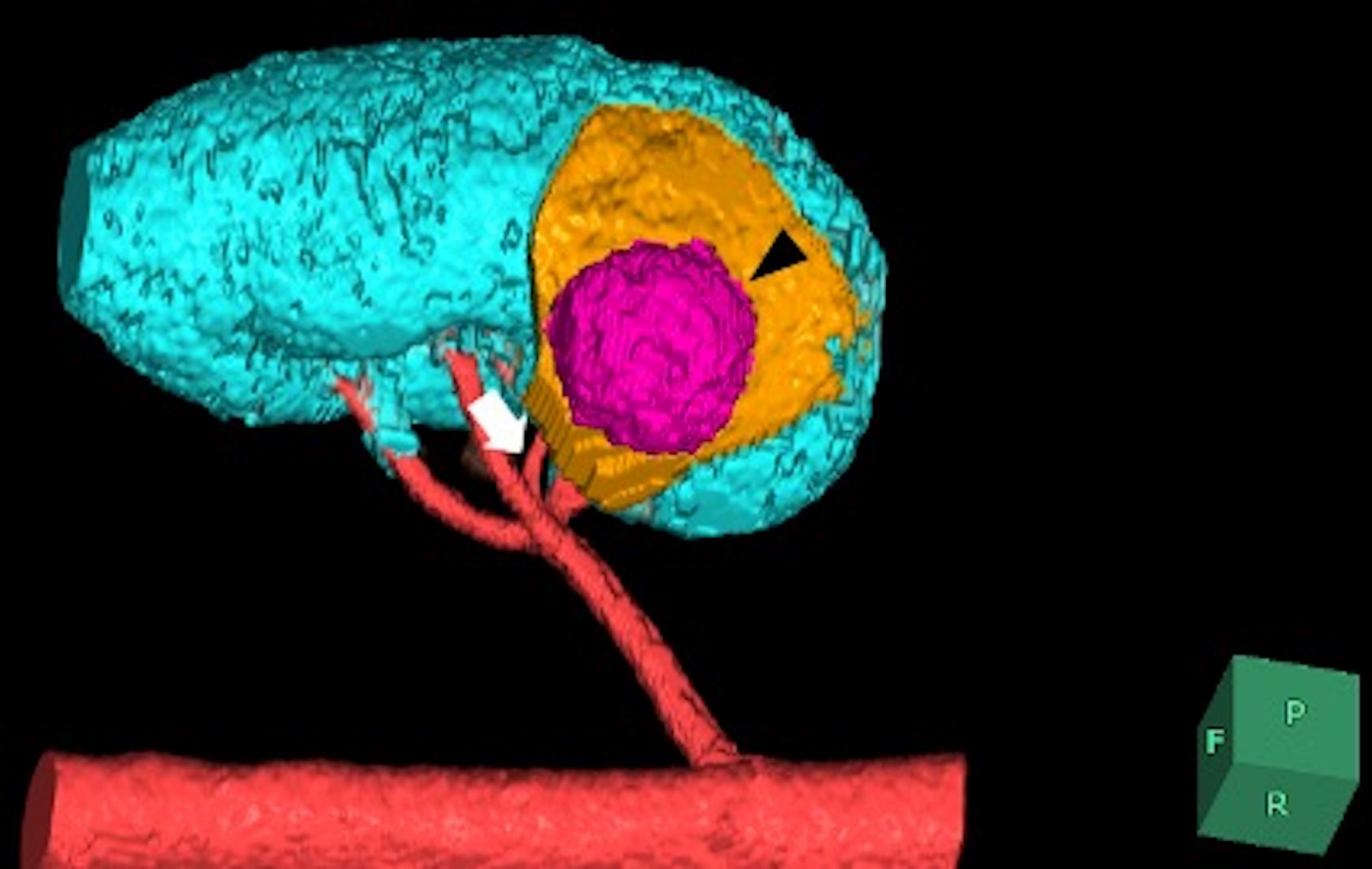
3D reconstruction of the CT scan. The renal tumor (black arrow) is seen on the dorsal of the kidney. The feeding branch (white arrow) entered the tumor directly from the first posterior branch of the renal artery and the ischemic area (yellow area) was identified.
